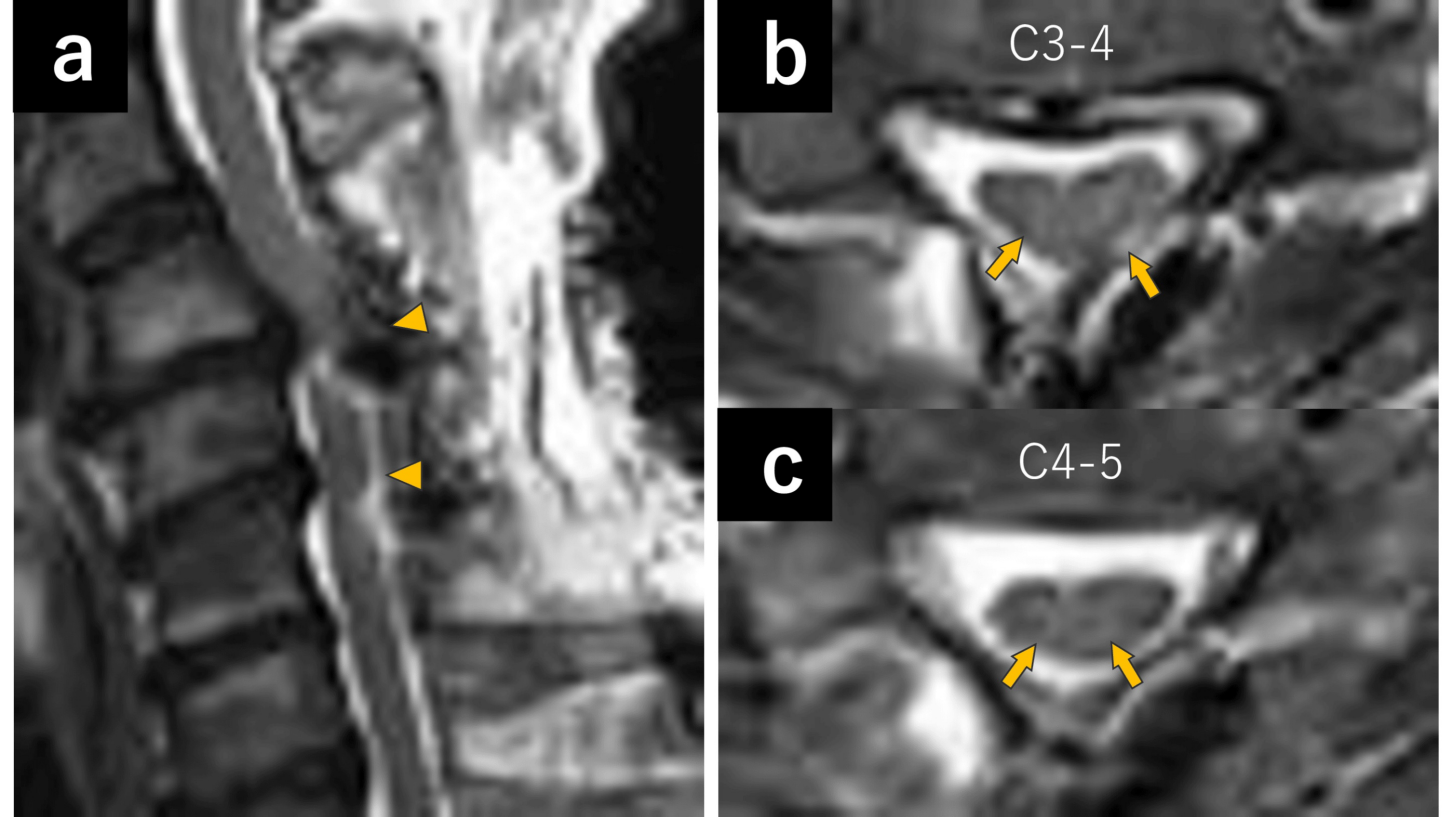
Postoperative cervical spine MRI findings a: sagittal T2-weighted MRI obtained one week after surgery showing adequate decompression at the C3–4 and C4–5 levels (arrowheads); b, c: axial diffusion-weighted MRI at the C3–4 and C4–5 levels demonstrating a slightly less distinct hyperintense area (arrows) than the preoperative images, consistent with the effects of decompression surgery.
